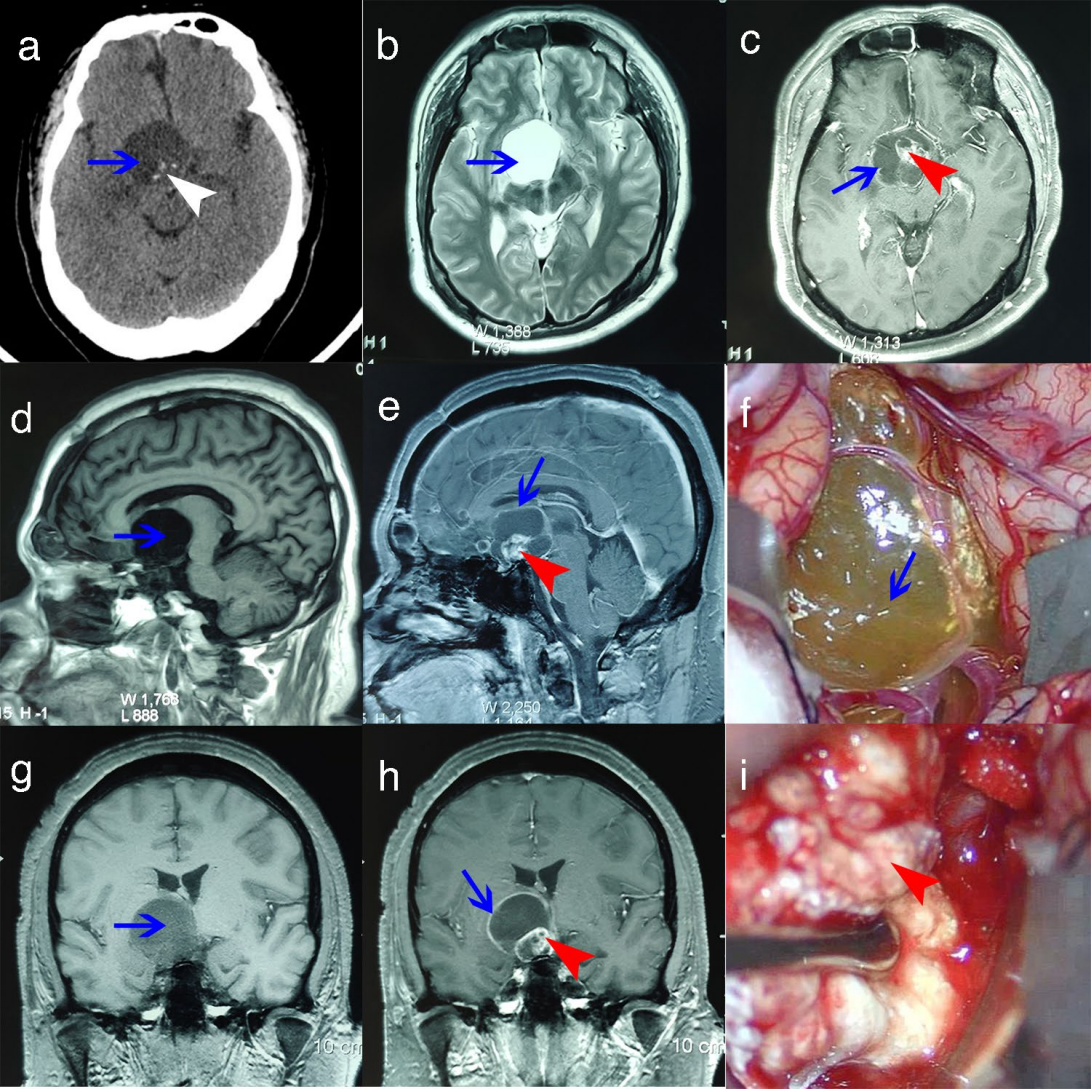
Preoperative and intraoperative imaging of cyst wall and solid body. (a) CT scan shows low-density cystic lesion and high-density calcifications (blue arrow, cyst; white arrowhead, calcifications). (b) T2-weighted axial MRI shows hyperintense signal lesion (blue arrow: cyst). (c,e,h) T1-weighted enhanced MRI shows the peripheral cyst wall and heterogeneous solid body (blue arrow, cyst wall; red arrowhead, solid body). (d,g) T1-weighted MRI shows hypointense signal lesions (blue arrow: cyst). (f,i) Cyst wall and solid body during operation (blue arrow, cyst wall; red arrowhead, solid part).
